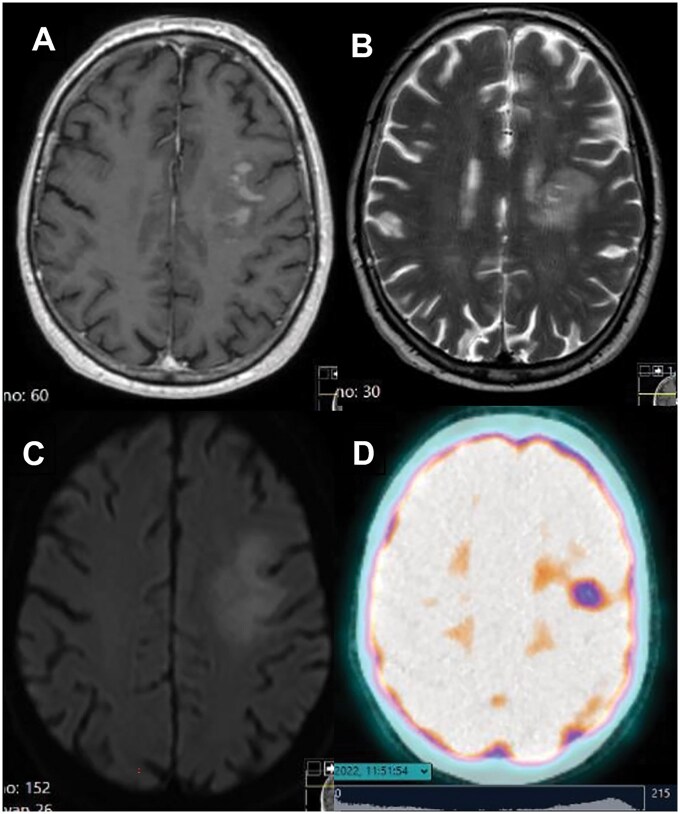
Multifocal primary central nervous system lymphoma in the left frontal and parietal lobe with (A) homogenous enhancement, (B) a low T2 signal, (C), diffusion restriction, and (D) FDG avid on the [^18^F]FDG-PET scan.
